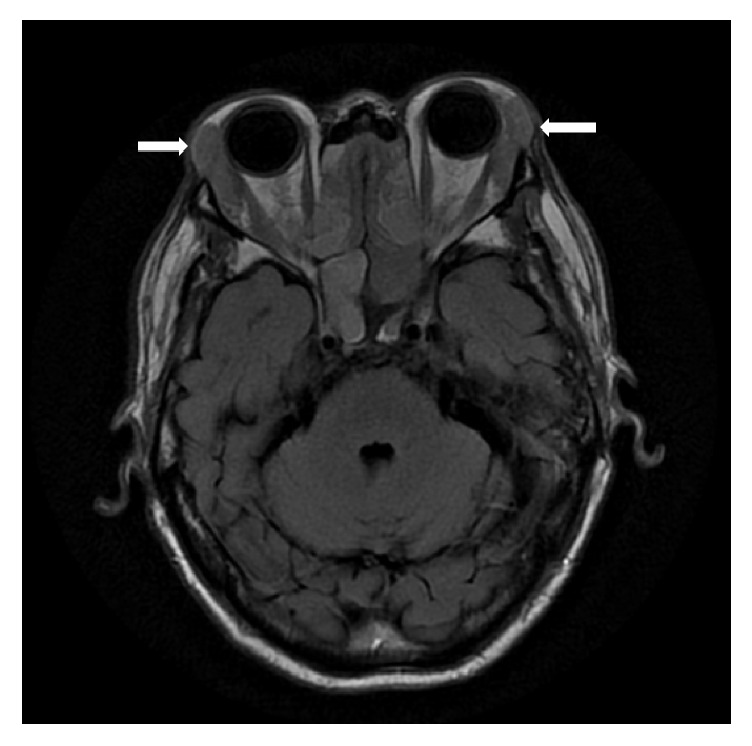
MRI brain showing bilateral enlargement (arrows) of the lacrimal glands.
